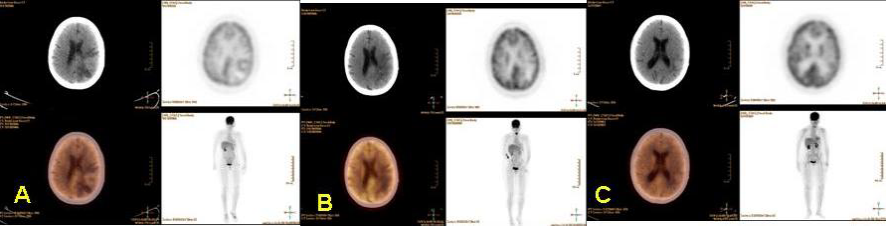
No extracranial FDG-avid lesions to suggest malignancy are seen by PET/CT. A) PET/CT, prior to craniotomy, showing anterior rim enhancement of the left parietal tumor. B) PET/CT revealing a photopenic area following second resection of the left parietal tumor. C) Follow-up PET/CT at 9 months.
